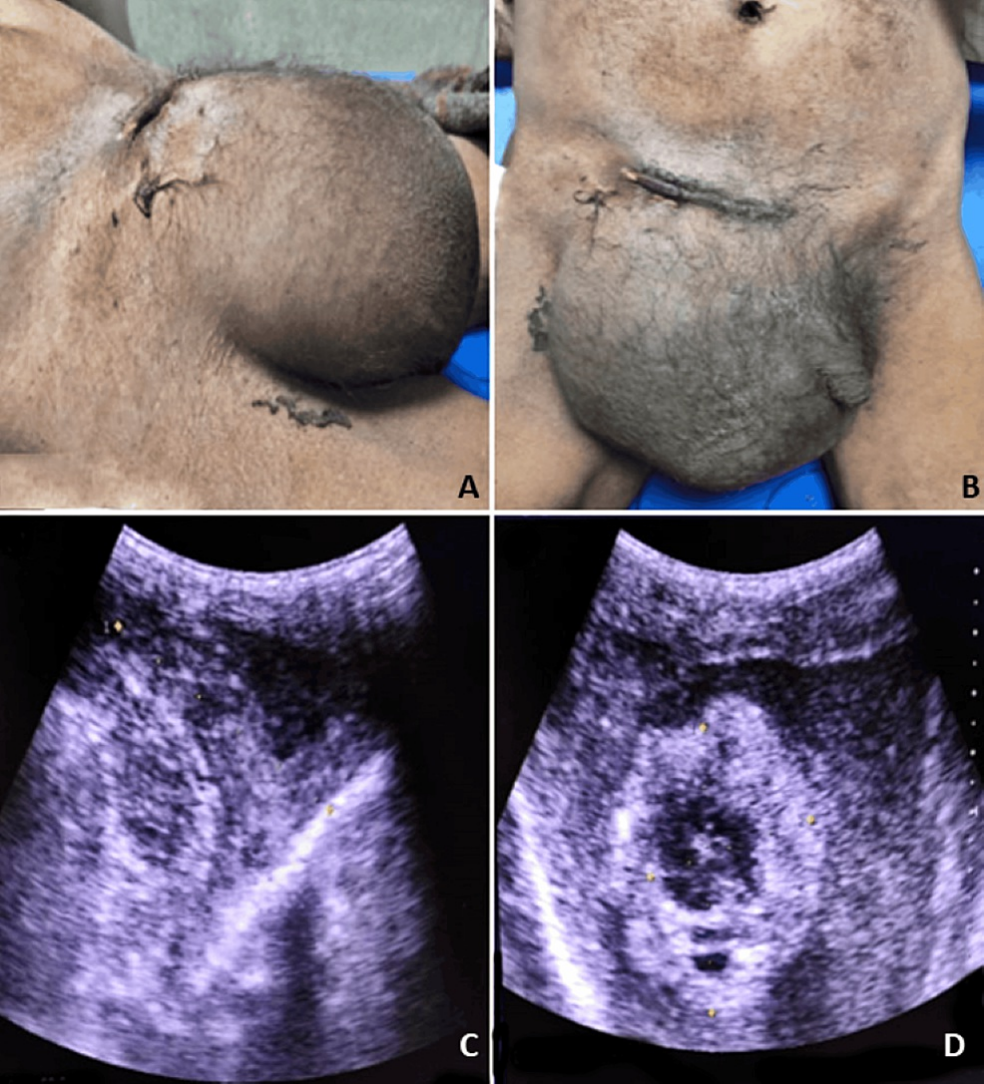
Giant right recurrent inguinal hernia immediately post-operation along with ultrasound images done on the same patient. (A, B) Gross appearance of recurrent inguinal hernia immediately post-operation; (C, D) ultrasound images of recurrent inguinal hernia immediately post-operation. Age: 42 years, sex: male.
